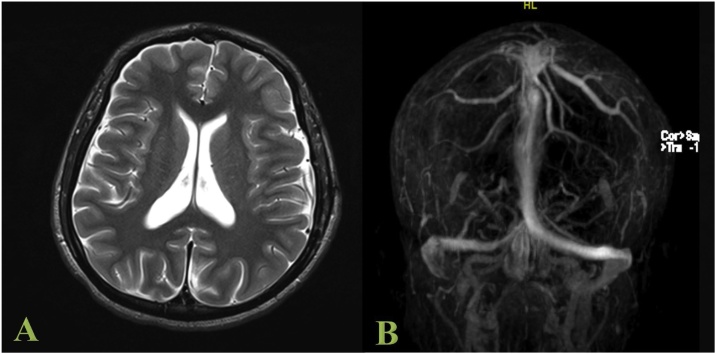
MRI (A) and MRV (B) brain performed six months following the second surgery for evacuation of SDH recollection showed complete resolution of the SDH, with residual partial thrombosis of the right sigmoid sinus; however, the sinus is patent and the venous flow restored.
